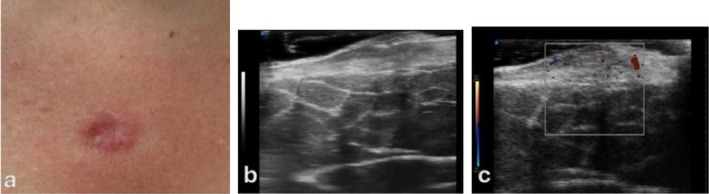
Representative HFUS findings in complicated healing patterns. (a) Clinical image of a hypertrophic/keloid scar (elevated, erythematous lesion). (b) B‐mode HFUS: Thickened, hyperechoic dermis with disrupted layering. (c) Colour Doppler: Marginal vascularity, indicating persistent cellular activity. HFUS distinguishes pathological scars from normal maturation by echogenicity, architecture and vascular profile.
